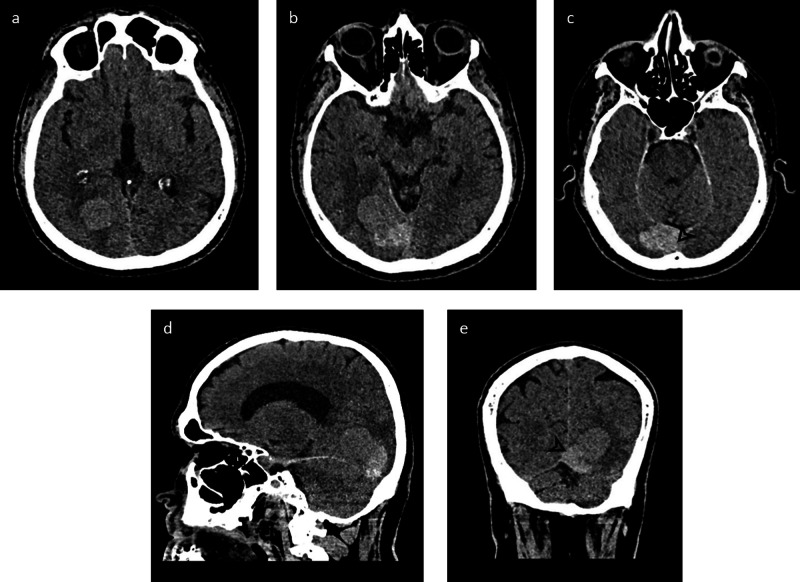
Non-contrast CT head Axial non-contrast CT head images along the superior (a), mid (b), and inferior (c) portions of the mass followed by sagittal (d) and coronal (e) reconstructions. There is a hyperdense mass, which is seen extending from the extra-axial space near the torcula along the posterior falx. There is mild surrounding vasogenic edema in the right occipital lobe. The mass exhibits a heterogeneous appearance with a relatively more dense component seen closer to its base with suspected dural venous sinus involvement (arrowhead) CT: computed tomography
